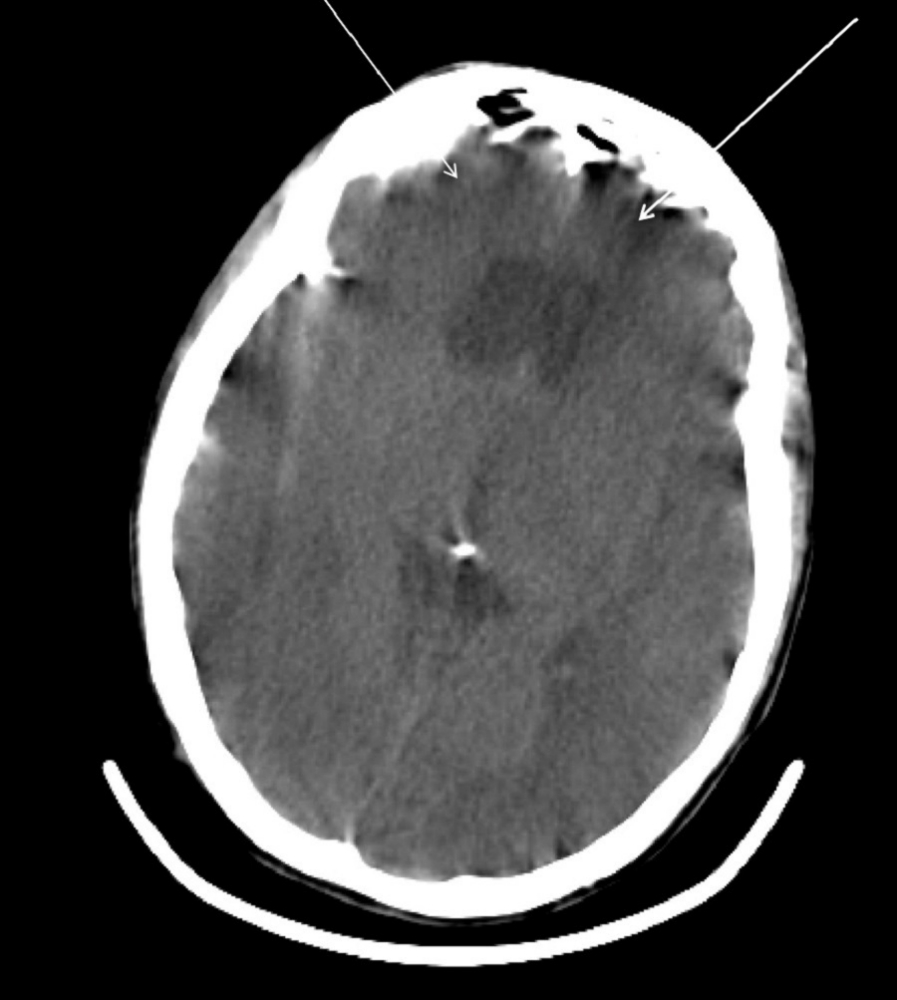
Postoperative head computed tomography (CT) without contrast demonstrating new acute infarcts involving the bilateral frontal lobes.
